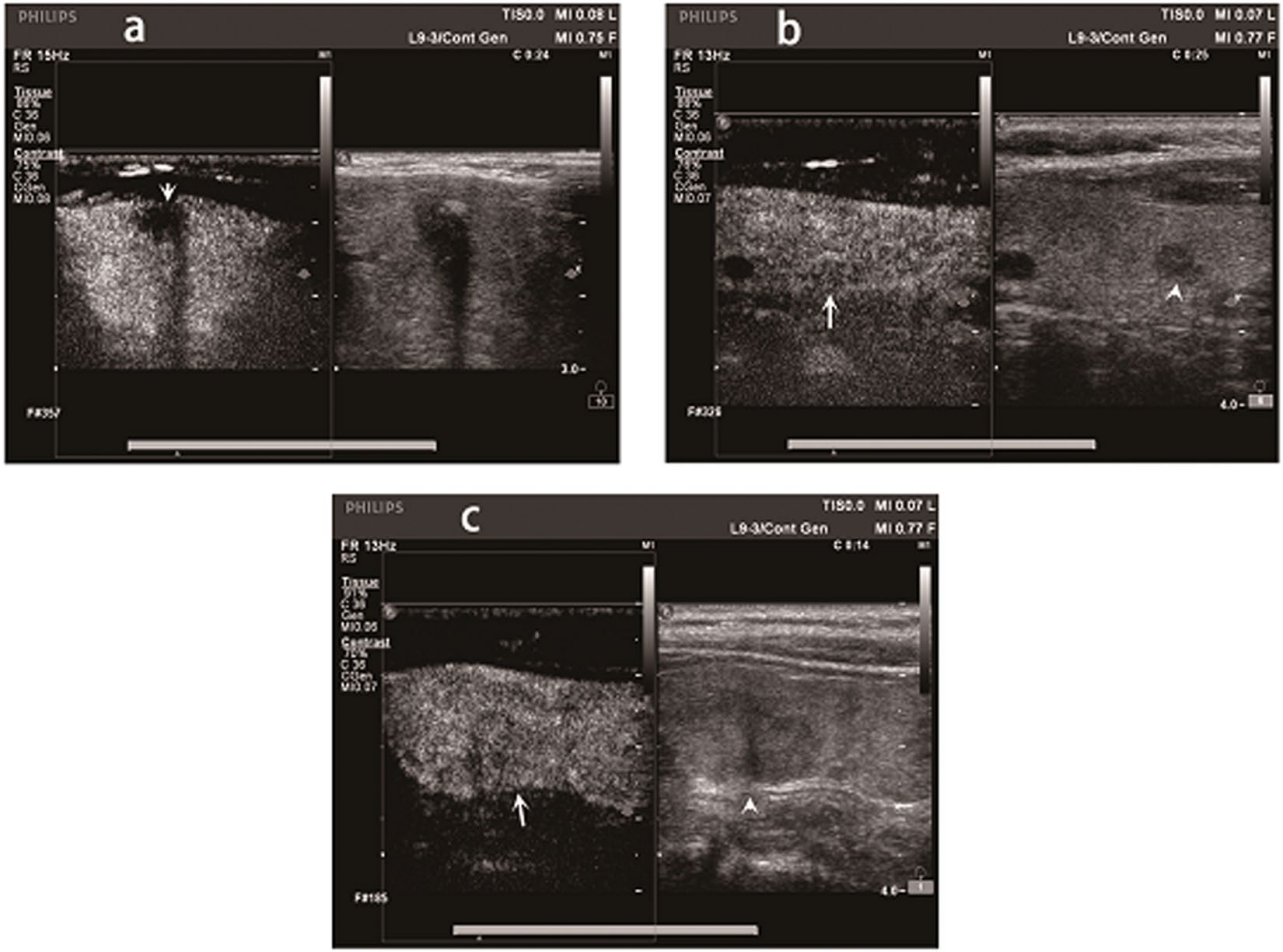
The Contrast-enhanced ultrasound (CEUS) images of different papillary thyroid carcinoma (PTC) patients with extracapsular extension (ECE). (a) CEUS image of a 48-year-old male PTC patient with ECE. The CEUS image clearly showed that the anterior thyroid capsular was invaded by PTC (arrow). (b) CEUS image of a 58-year-old female PTC patient with ECE. The nodule showed by arrow head was the PTC nodule. ECE was not clearly confirmed by CEUS (arrow). (c) CEUS image of a 50-year-old female PTC patient with ECE. The nodule showed by arrow head was the PTC nodule. ECE was not clearly detected by CEUS (arrow).
